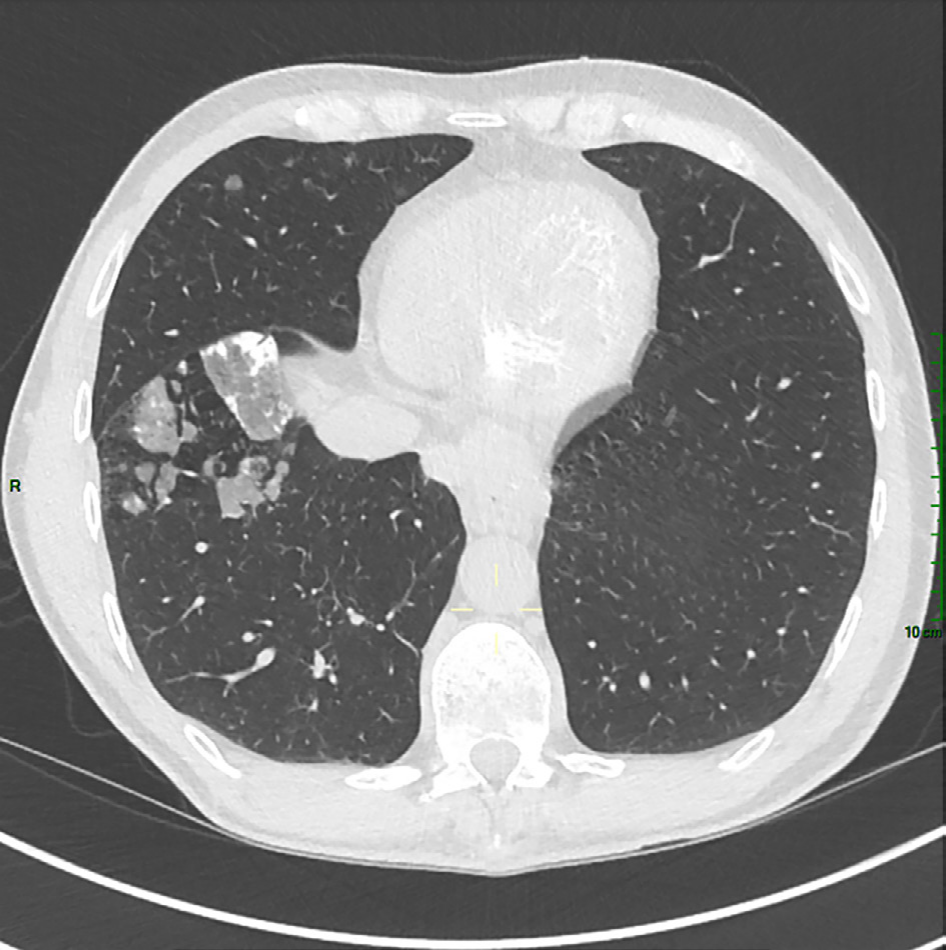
Axial chest CT: ground‑glass nodular opacities, partially calcified.
